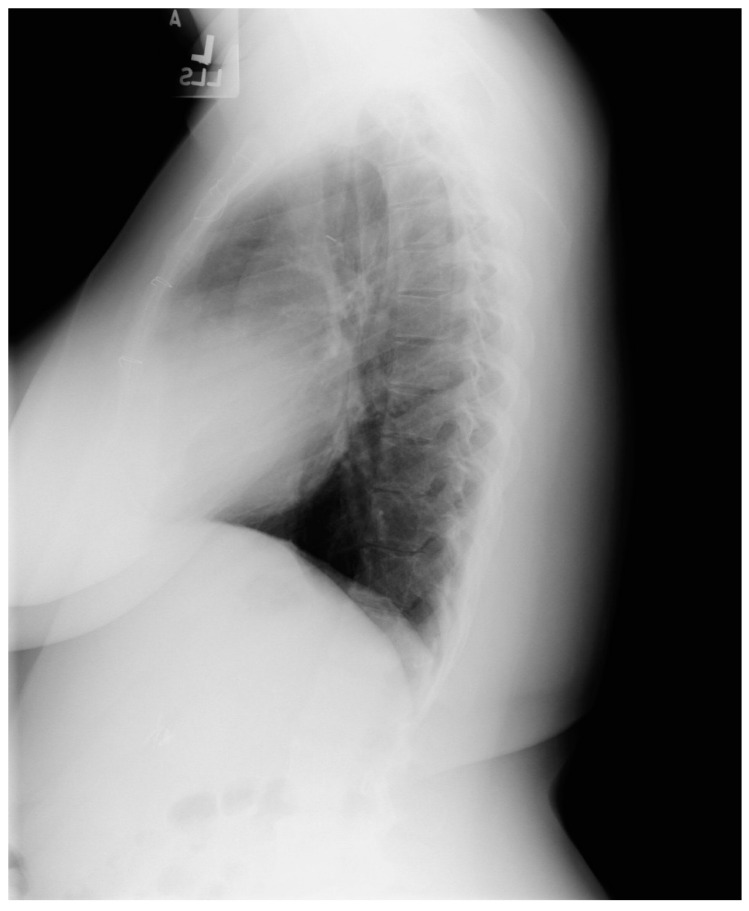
Lateral chest X-ray in an adult with previously operated tetralogy of Fallot (toF) above: This X-ray shows sternal wires from a previous median sternotomy. The retrosternal airspace is mildly occupied by right ventricular enlargement. There is a metallic surgical clip in the upper middle mediastinum from one of her previous surgical procedures. Doppler echocardiography revealed severe pulmonary valve regurgitation, moderate–severe tricuspid regurgitation, moderate–severe right ventricular dilation and dysfunction. The patient subsequently underwent pulmonary valve replacement and tricuspid valve repair.
